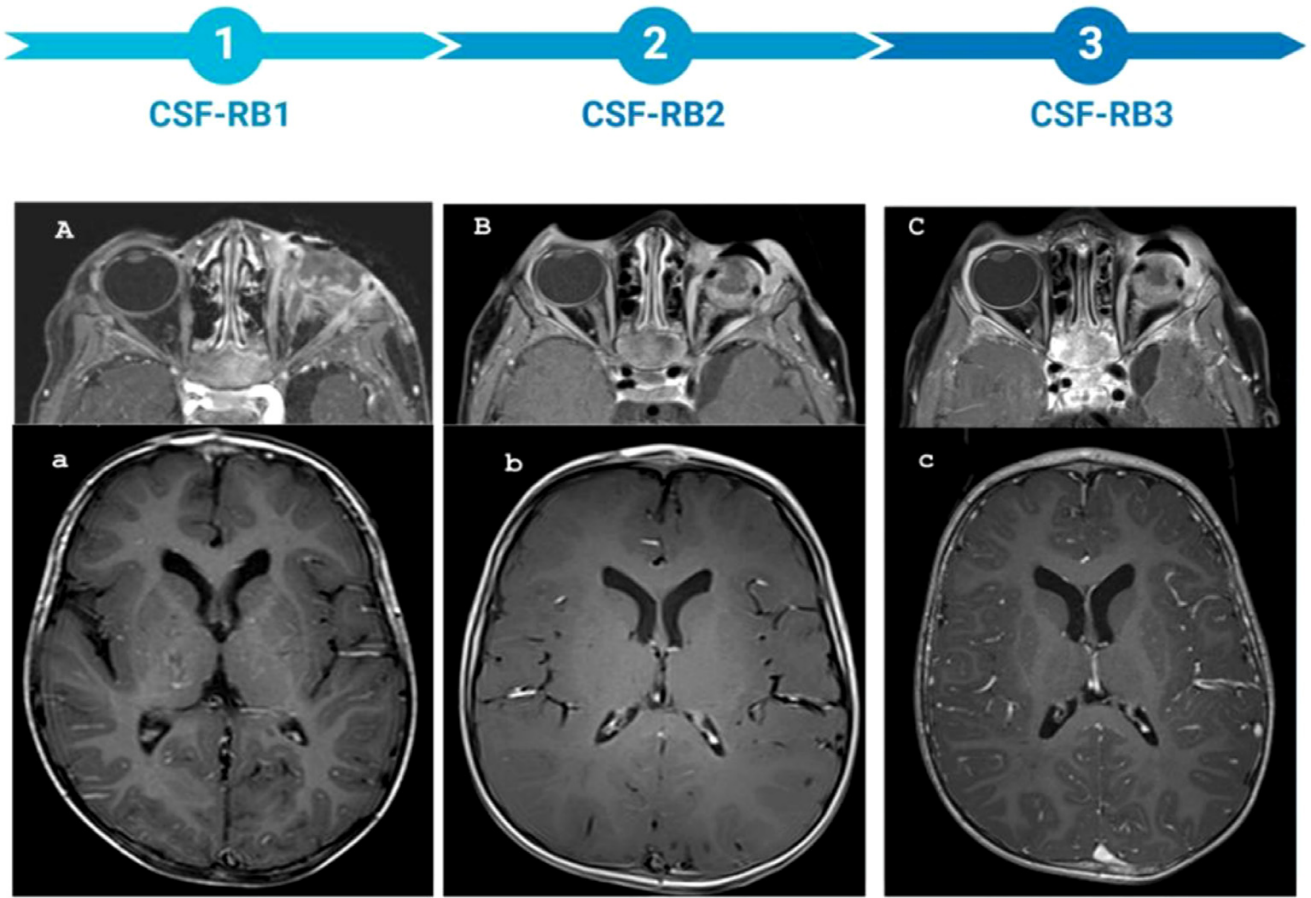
MRI of the patient throughout the entire treatment, along with the corresponding sample time points. (A–C) axial DIXON T1 post-CM: the timeline of optic nerve involvement. (A) presence of pathological tissue localized in the left retro bulbar site. This tissue takes the contrast medium (MDC) which localizes in the left retro bulbar region, is characterized by irregular profiles and extends to partially involve the extrinsic muscles, making their distal portion difficult to identify. The retro bulbar segment of the optic nerve is also partially affected, showing pathological enhancement. Additionally, there is pathological enhancement of the periorbital soft tissues. (B) Extensive involvement of the left optic nerve is evident following enucleation (C) Further progression of optic nerve involvement is observed, with irregular profiles of optic nerve. (a–c) axial T1w pst-CM: timeline of meningeal involvement. (a) no meningeal enhancement, (b) no meningeal enhancement, (c) intense meningeal enhancement.
